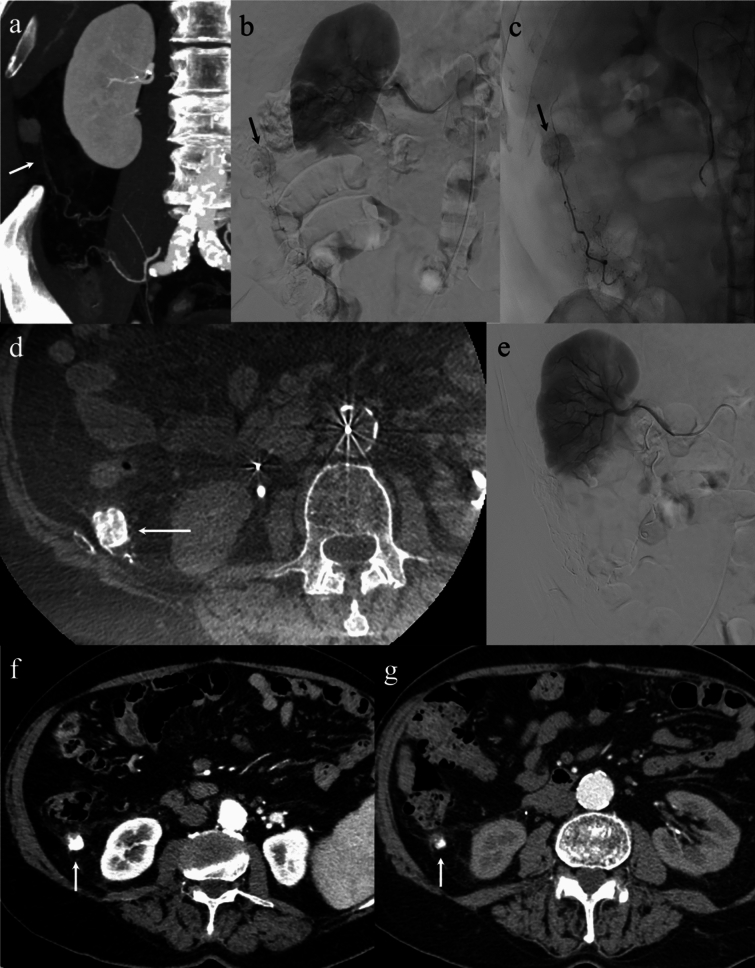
Preliminary evaluation of maximum intensity projection (MIP) of computed tomography (CT) images allowed accurate delineation of the tumor-feeding vessel of the peritoneal metastasis from hepatocellular carcinoma (HCC), which originated from a thin branch of the right renal artery (arrow in a). The angiographic study confirmed the blood supply of the extrahepatic metastasis and its rich blood supply similar to its counterparts in the liver (arrow in b and c). The cone-beam computed tomography (CT) also demonstrated the superselective catheterization of this thin tumor-feeding artery and its limited supply to the abdominal parenchyma and intestine (arrow in d). After transarterial chemoembolization (TACE), the occlusion of the tumor-feeding vessel is demonstrated together with the patency of the remaining hepatic arteries (e). Contrast-enhanced CT performed 1-month post-TACE revealed successful therapeutic agent delivery and the disappearance of arterial enhancement (arrow in f). The Lipiodol deposition was reduced at the 12-month follow-up, and the lesion demonstrated a significant dimensional regression (arrow in g)
